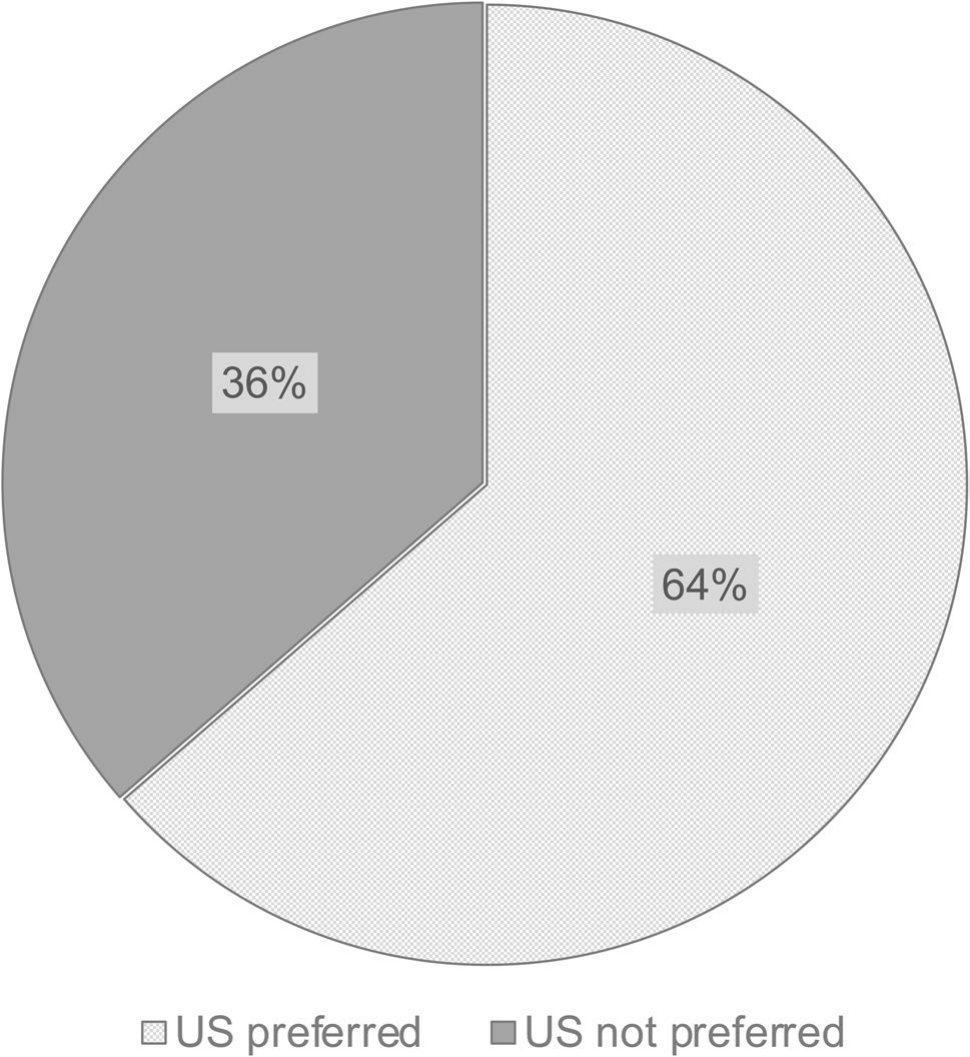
The role of ultrasound in defining anatomy and planning liver transplantation in children: Insights from 22 pediatric liver transplantation centers *US* ultrasound
